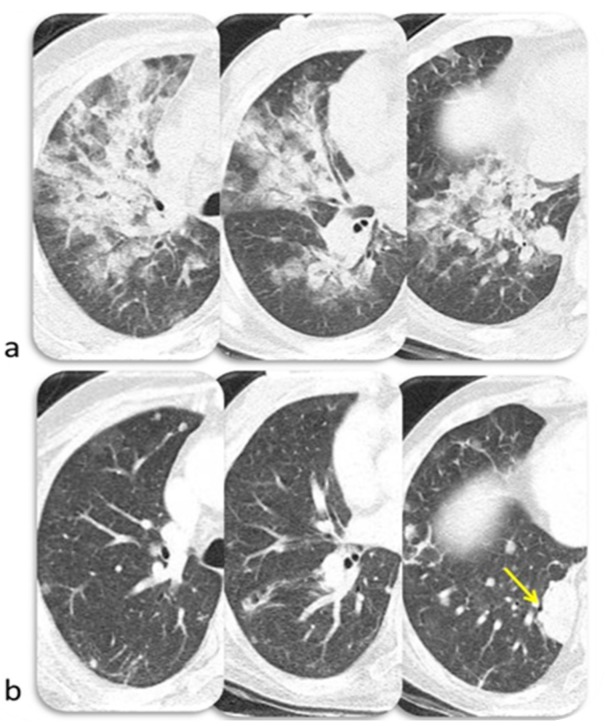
Immune related pneumonitis (pattern AIP/ARDS) in a 47-year-old woman with metastatic thymic carcinoma during treatment with pembrolizumab (a). CT image after 2 months of steroid therapy shows resolution of ARDS (b) and progressive disease (yellow arrow). © Department of Radiology, University of Pisa.
